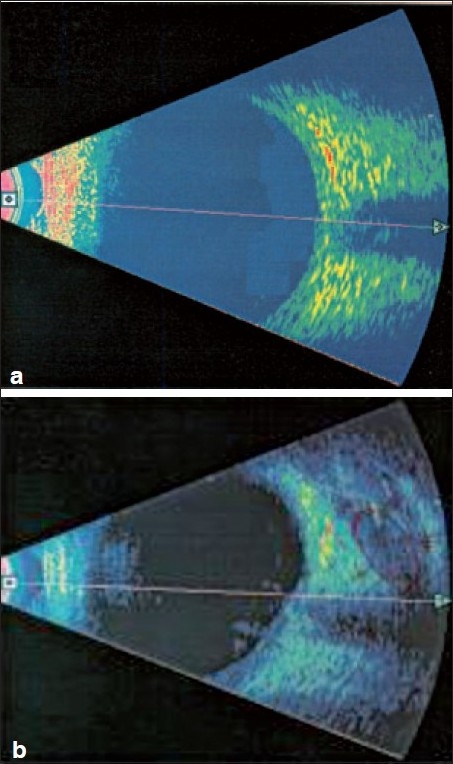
(a) The ultrasound axial scan through the optic nerve preinjection with homogenous echogenecity of retro orbital path around the optic nerve. (b) The ultrasound lower vertical scan 10 minutes after injection and the axial scan pass below the red ring that demonstrate the hypo-echogenic pattern of local anesthetic fluid collection in the inferior surface of the nerve which starts to track in the direction of the muscle cone
